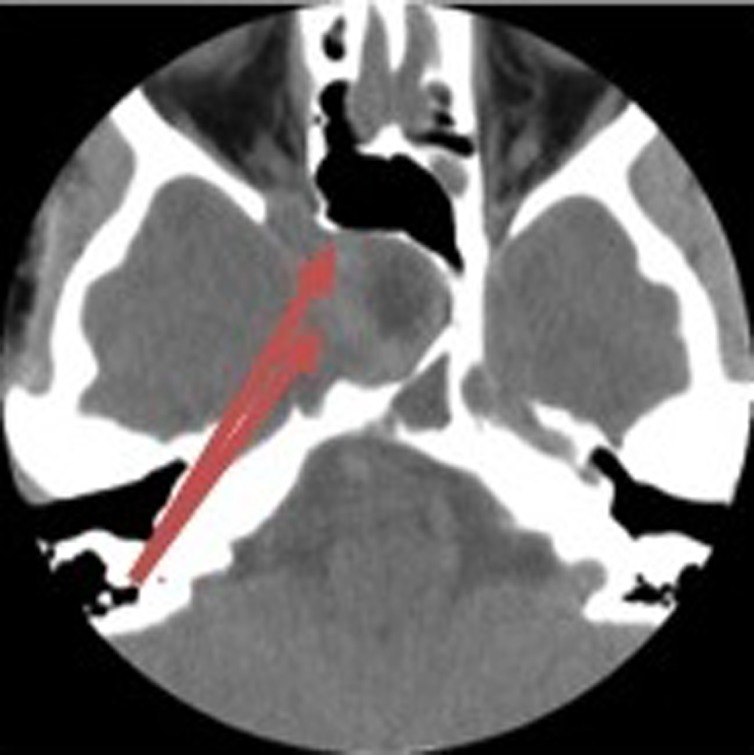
Computed tomography of the pituitary: arrows highlight areas of thinned and deficient bony sella.
